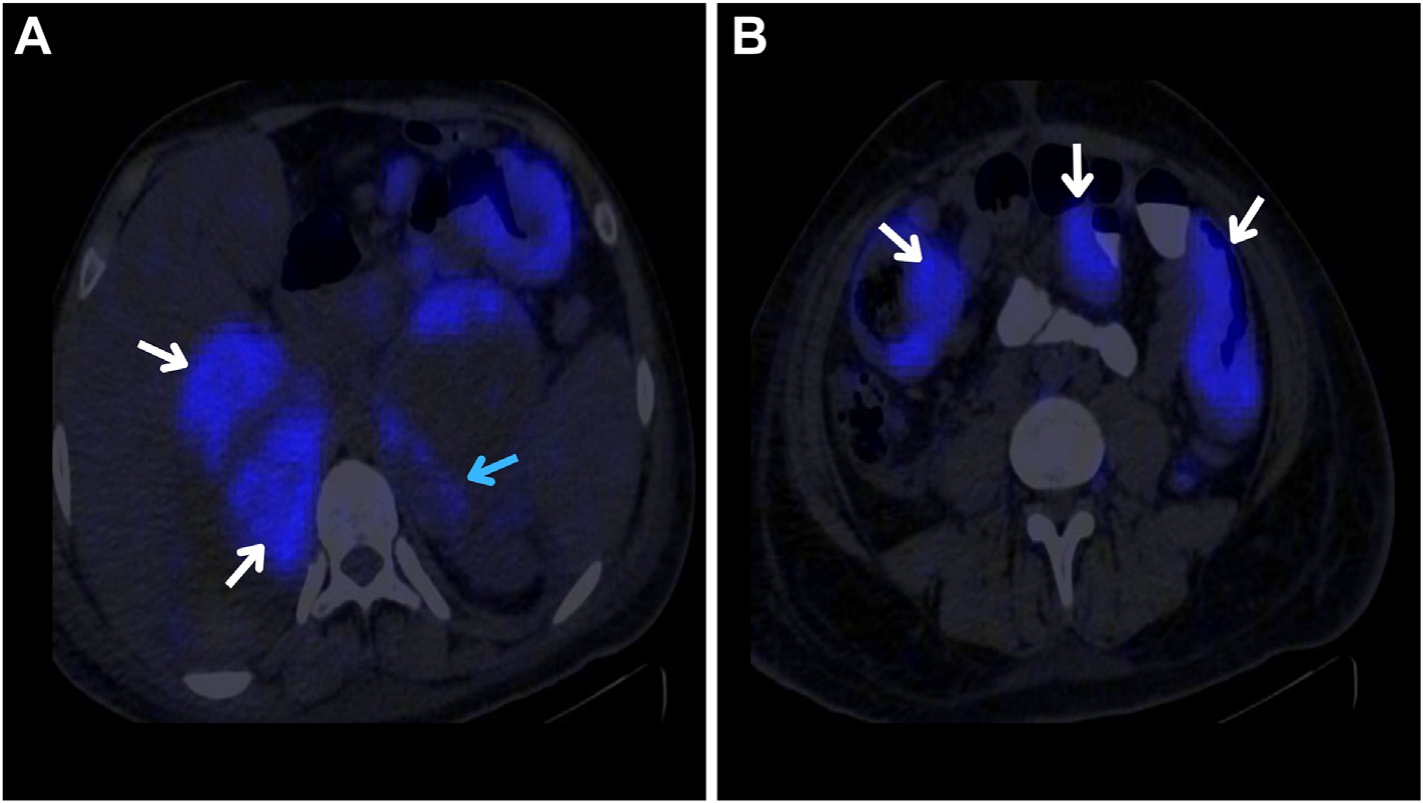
Positron emission tomography–computed tomography imaging of the abdomen in an axial plane. *A*, Fluorodeoxyglucose avid uptake was observed in peripheral dense nodules of adrenal hematomas (white arrow) and in multiple enlarged para-aortic lymph nodes (blue arrow). *B*, Fluorodeoxyglucose avid uptake was noted in diffuse wall thickening involving segments of small bowel loops (white arrow).
